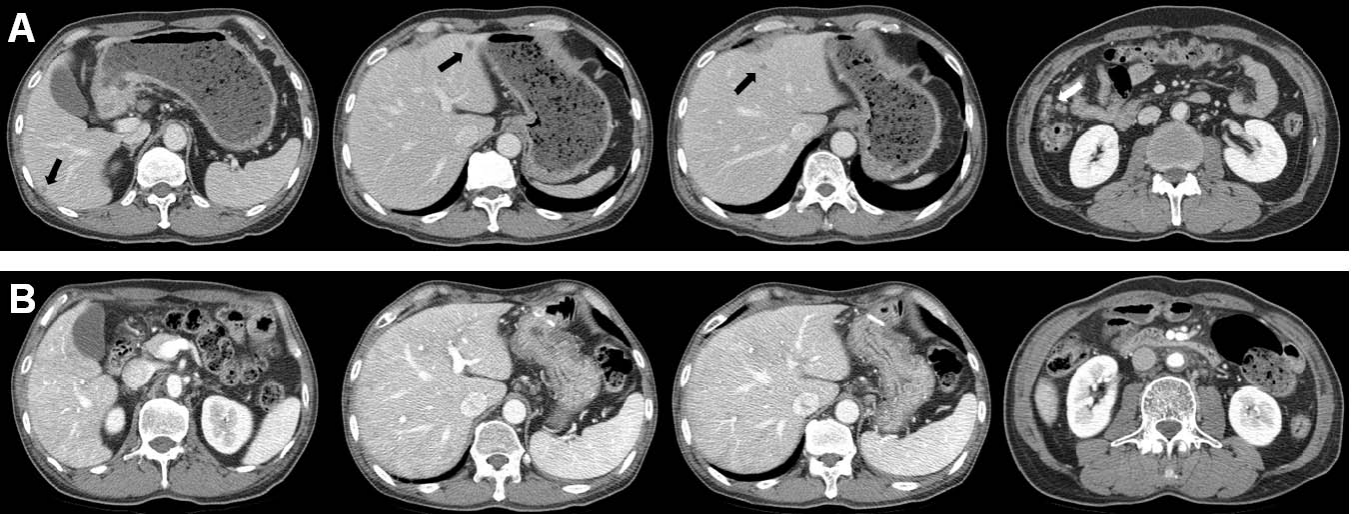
Computed tomography (CT) scan (A) before and (B) after chemotherapy. (A) A CT scan showed thickening of the gastric wall, adjacent fat infiltration, and a large amount of food in the stomach, suggesting a passage disturbance. Lymph nodes along the common hepatic and left gastric arteries were markedly enlarged, and multiple enhancing omental masses (white arrow) and nodular peritoneal thickening, suggesting peritoneal carcinomatosis, and multiple hepatic metastases (black arrows) are shown. (B) Complete disappearance of thickening of the gastric wall, the metastatic foci in the liver, omental infiltration of metastatic nodules, and enlarged lymph nodes were observed.
